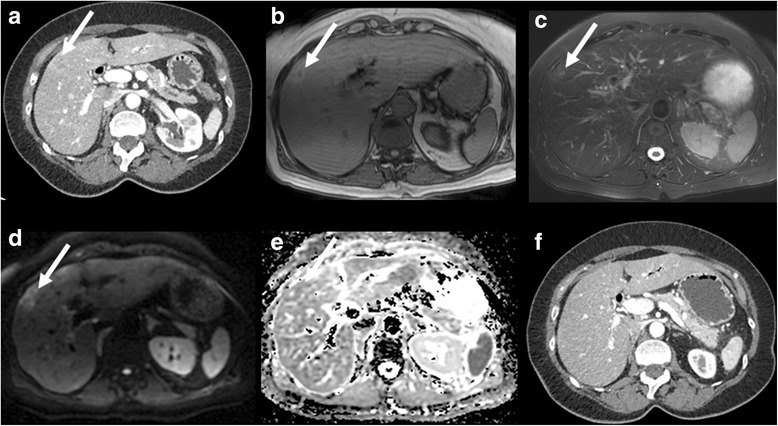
Case 5. CECT and MRI demonstrate a peripheral hypovascular lesion, surrounded by THAD (a), with high SI on T1WI (b) and T2WI (c), as well as restriction on DWI (d, e). The lesion resolved after 4 months of follow-up (f)
